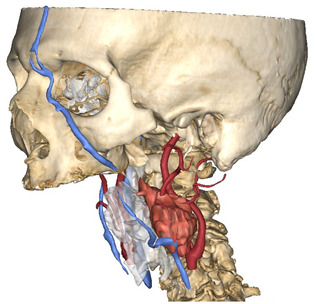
Single cervical metastasis from squamocellular carcinoma of the oral oral cavity	CT MR: VIBE TOF VEN3D NNV	Microsoft Hololens 2
